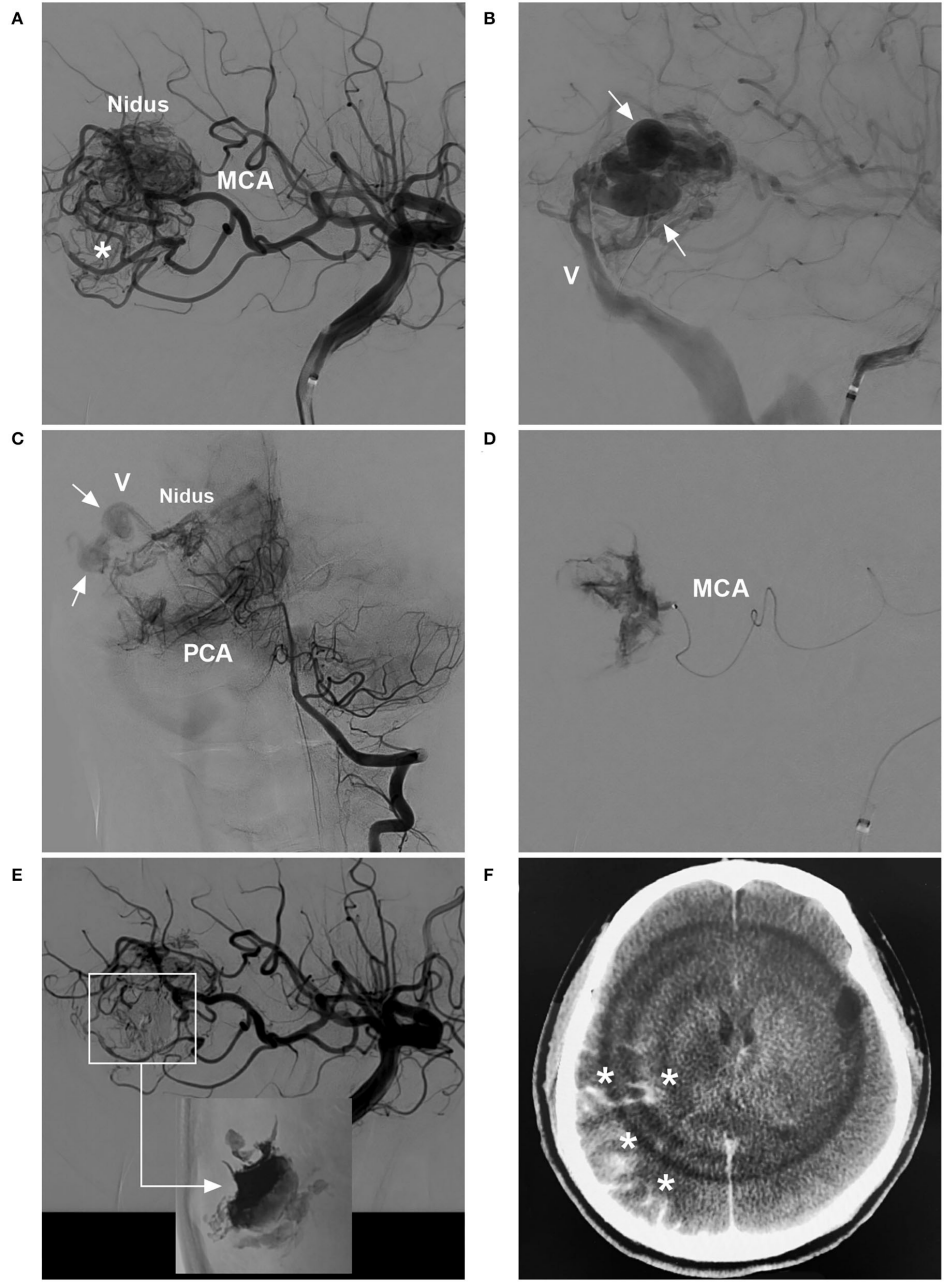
BAVM with intraoperative nidus perforation (case 12). (A) Arterial-phase angiogram of the ICA showing the BAVM supplied by the MCA. (B) Late arterial-phase angiogram of the ICA showing the draining vein (V) with venous aneurysms (arrows). (C) Arterial-phase angiogram of the VA showing the BAVM also supplied by the PCA. (D) Selective microcatheter angiogram showing contrast agent extravasation during catheterization and penetration of the nidus. (E) Arterial-phase angiogram of the ICA showing the prevention of contrast agent extravasation by continuous embolization (frame); Onyx casting (right-angled arrow) without embolization of the nidus (inset). (F) X-per CT showing contrast agent extravasation (asterisks) in subarachnoid space. BAVM, brain arteriovenous malformation; CT, computed tomography; ICA, internal carotid artery; MCA, middle cerebral artery; PCA, posterior cerebral artery; VA, vertebral artery.
